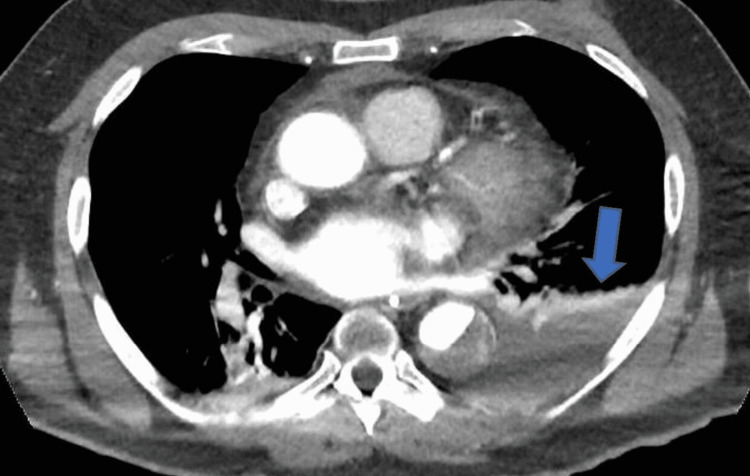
Computed tomography pulmonary angiography scan showing large left-sided haemothorax as shown by the pointed arrow
